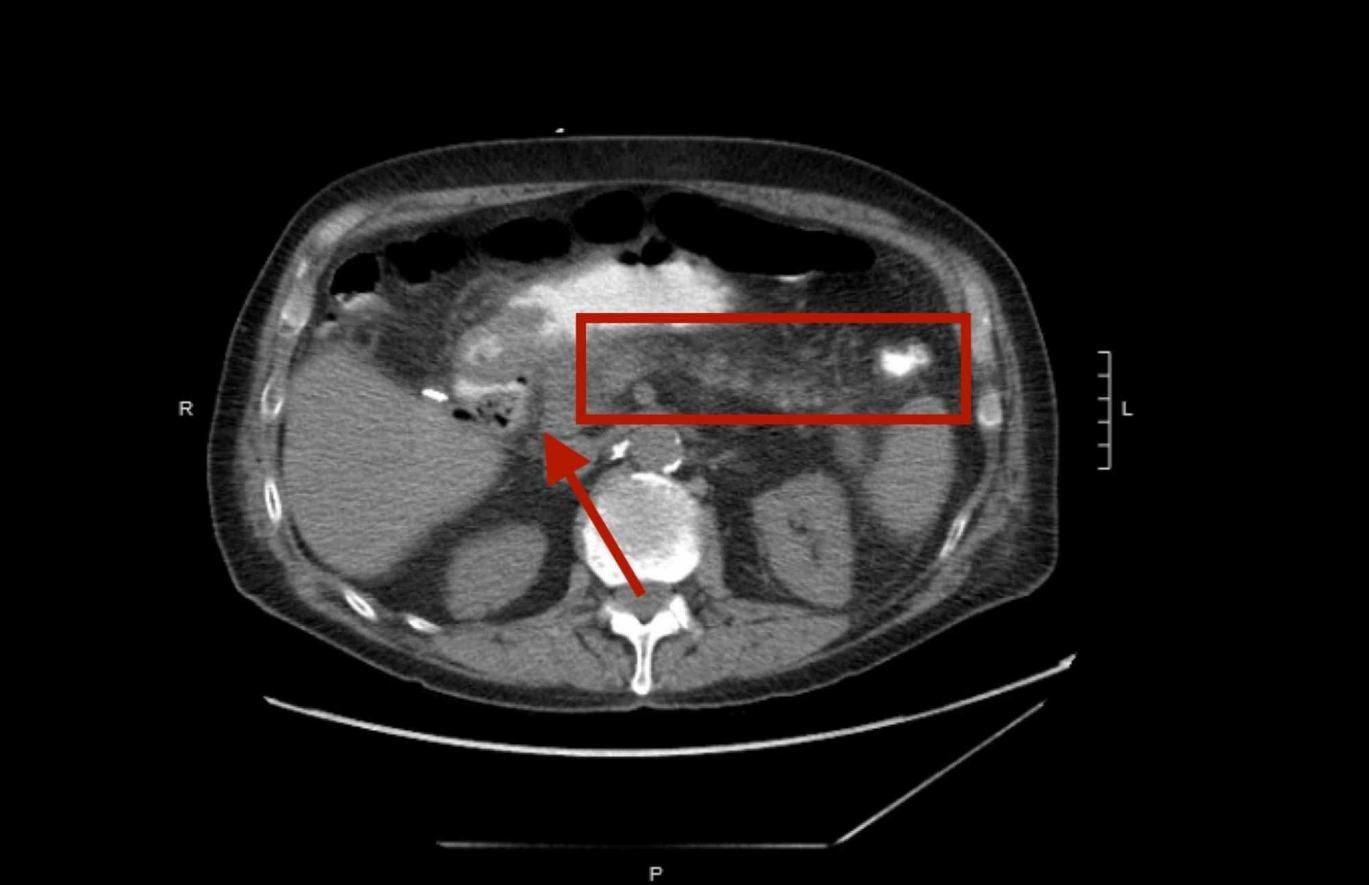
CT abdomen and pelvis without contrast
